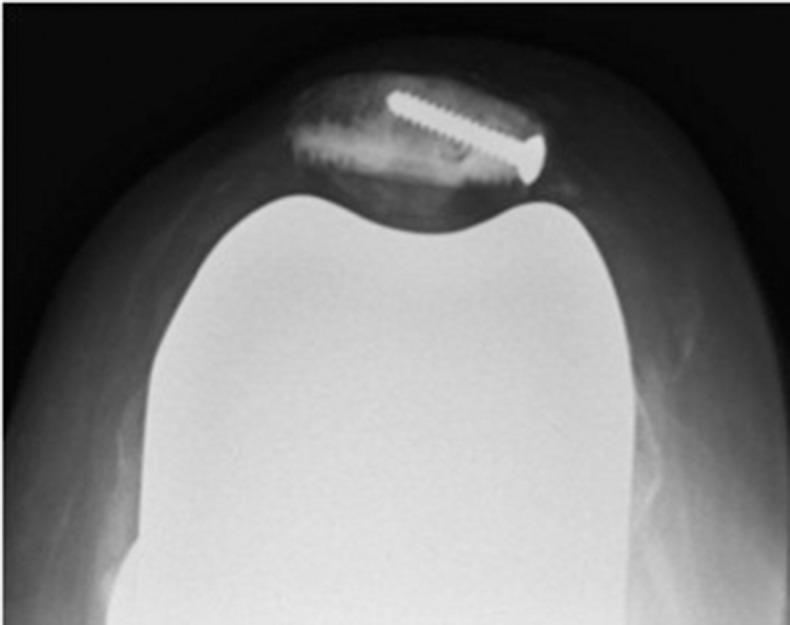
Radiography of the reconstructed patella at five-year follow-up appointment
